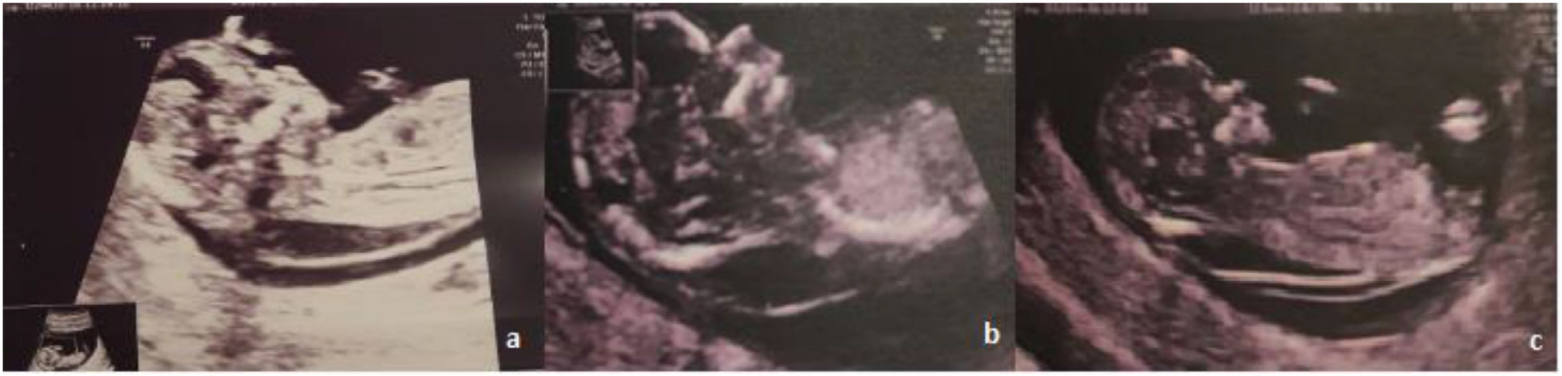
Ultrasonographic images of the fetus.
